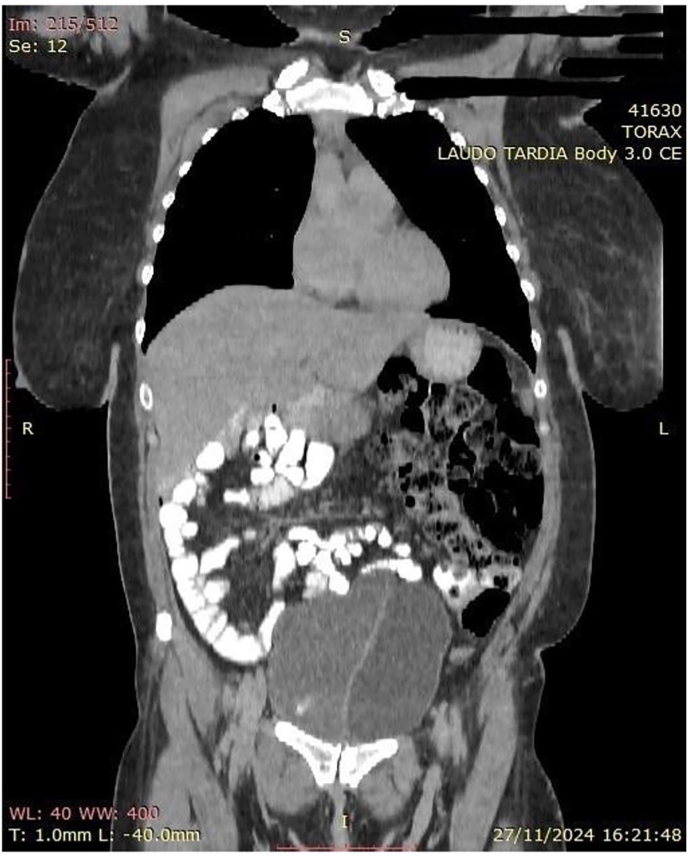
Computed tomography, coronal plane. Loops of the large intestine on the left; loops of the small intestine on the right; two bladders.
